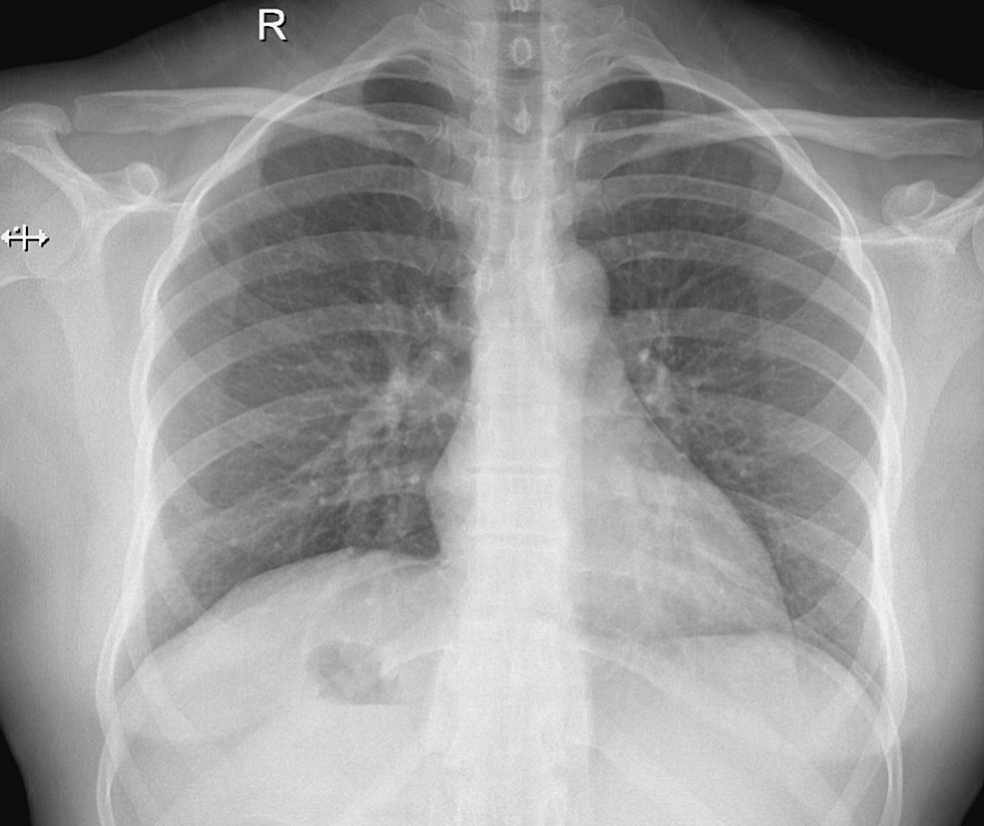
Chest x-ray images of the patient with situs inversus partialis. Gastric gases were noted under the diaphragm on the right side while the heart elements were in their natural positions.
